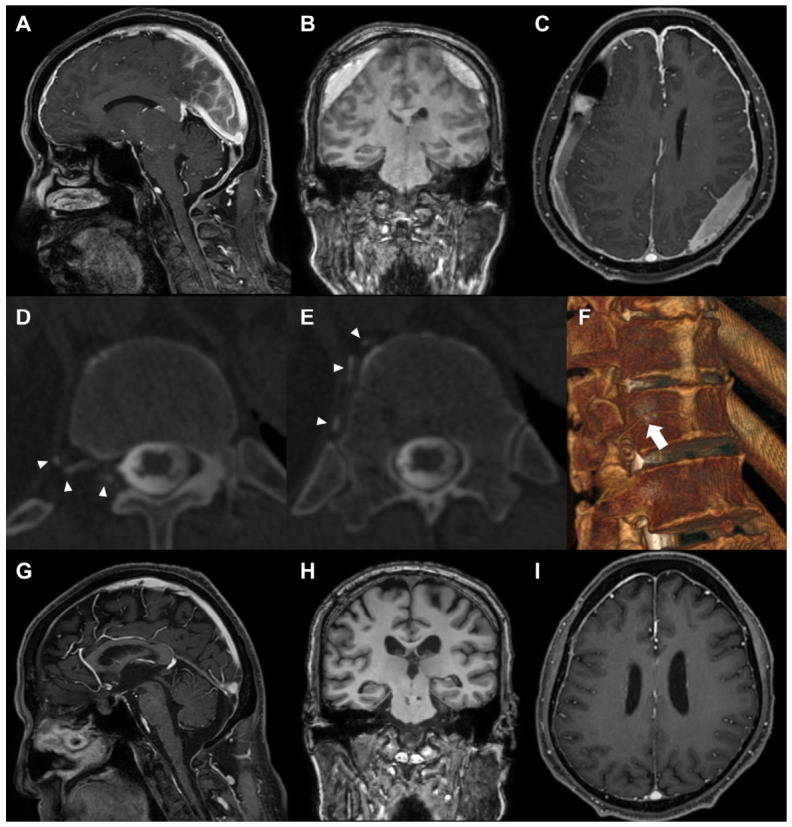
A case of 67-year-old male with Type 3 leak. (A–C) Preoperative brain MRI demonstrating a bilateral chronic subdural hematoma, pachymeningeal enhancement, and venous engorgement, with a Bern score of 9. (D,E) Lateral decubitus CT myelography revealing abnormal contrast egress from the right T12–L1 diverticulum into the paraspinal vein, consistent with a CSF–venous fistula. The white arrowheads indicate the draining paraspinal vein opacified through the CSF–venous fistula. (F) Three-dimensional reconstructed image showing the fistula (white arrow). (G–I) Postoperative brain MRI demonstrating a Bern score of 2 and complete resolution of the subdural hematoma.
